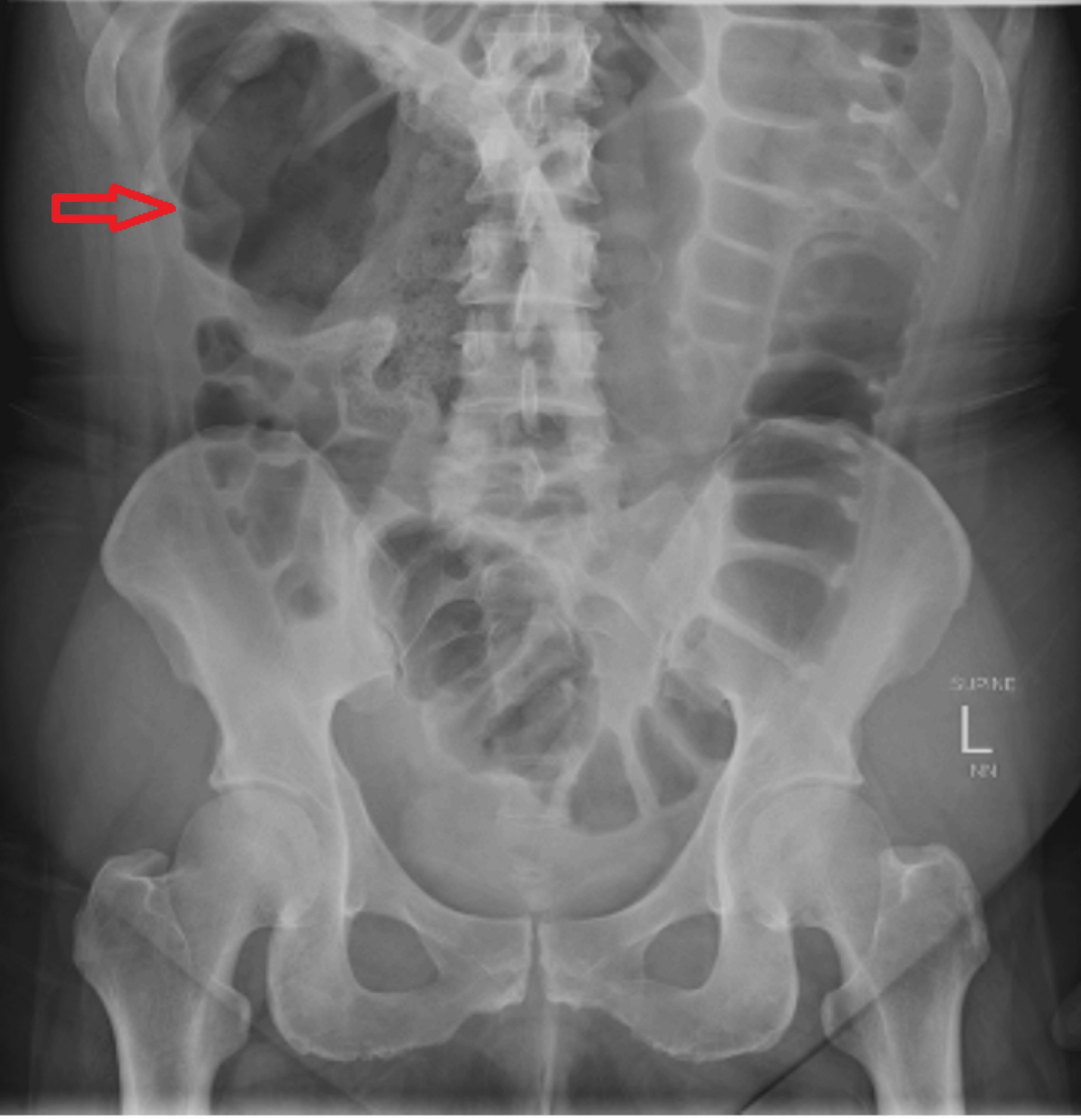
Abdominal X-ray demonstrating increasing colonic dilation (red arrow).
